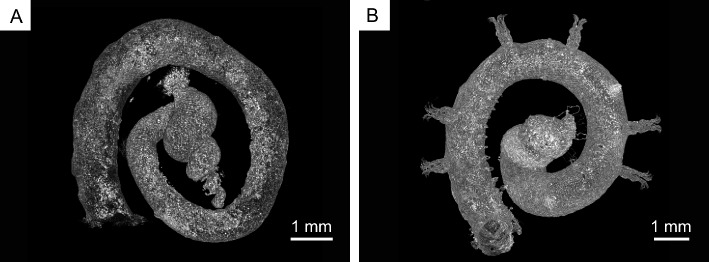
Micro X-ray CT photometry view of the orifice tunnel in *Heterocyathus* sp. A showing a circular to oval single aditus leading to a horizontally curved cylindrical tunnel (not spiral) running nearly parallel to the gastropod shell external surface. (A) Tunnel diameter increasing gradually from proximal to distal sides. (B) Tunnel diameter decreasing gradually from proximal to distal sides.
